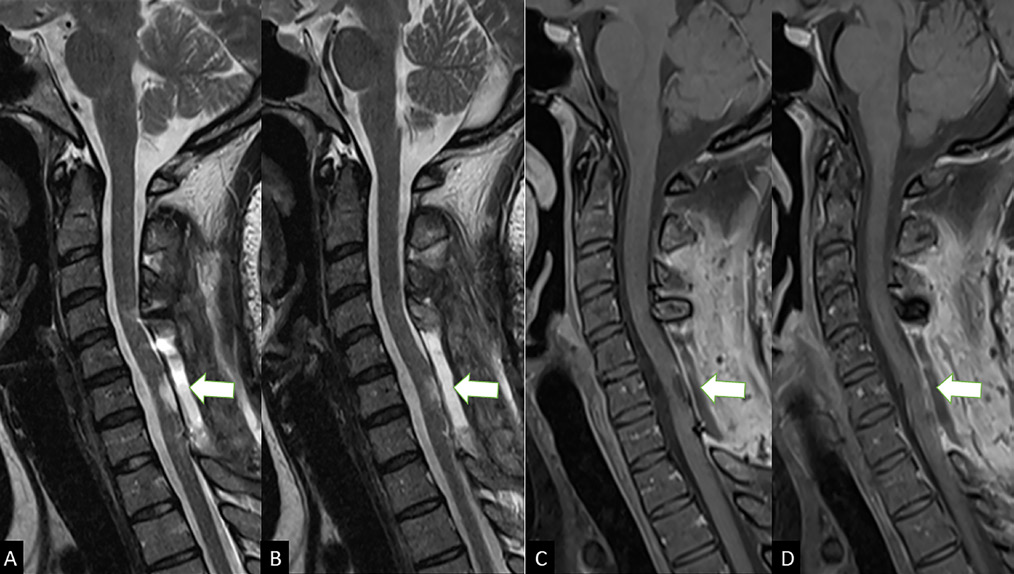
Conventional sagittal MRI images on *T*_2_WI
